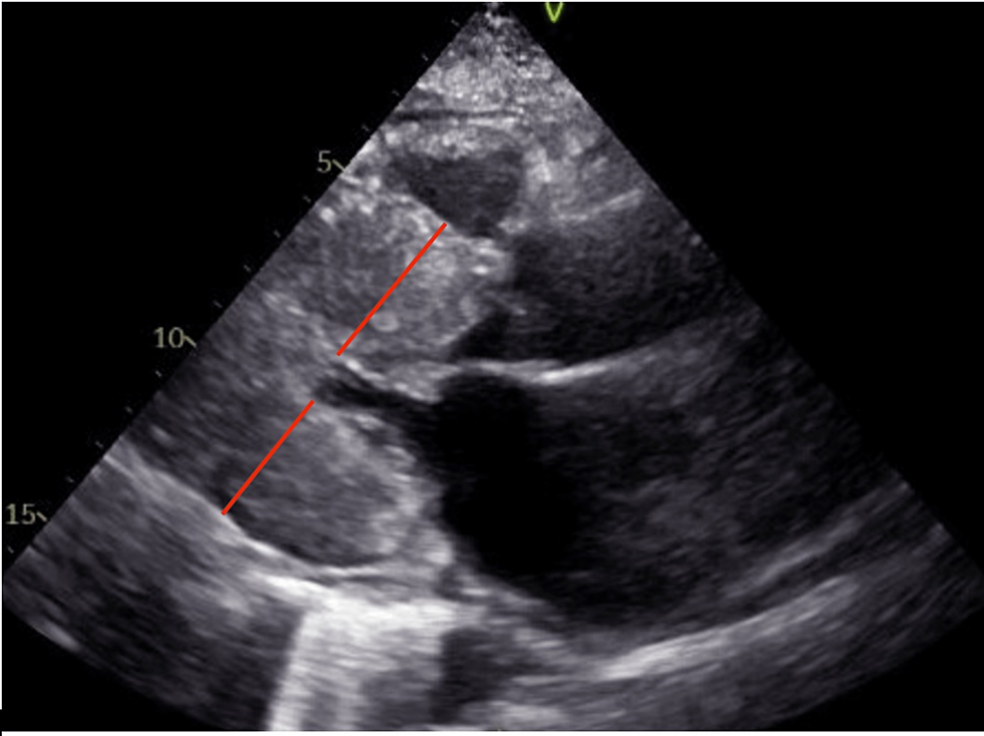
Echocardiogram Red lines show the width of the left ventricular wall
